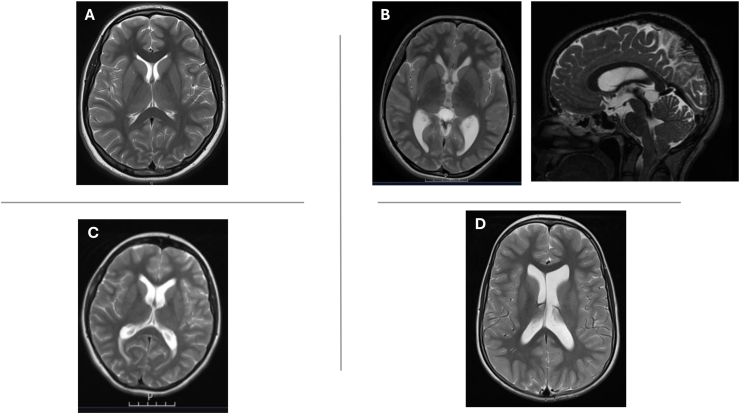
Preoperative MRI scans of four patients who later developed postoperative communicating hydrocephalus following posterior fossa decompression surgery. None of the patients exhibited clinical signs of hydrocephalus preoperatively. Retrospective imaging review revealed subtle findings such as borderline ventriculomegaly (B, C and D) suggesting altered CSF dynamics.
